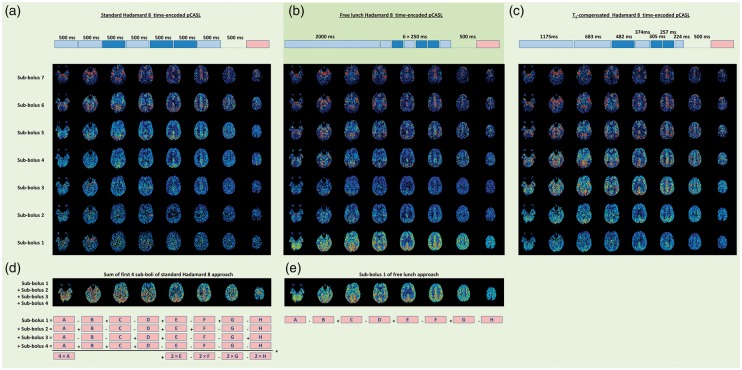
The result of three Hadamard time-encoded, background suppressed pCASL-scans of 5.5 min each (scans acquired on a 3 T MRI scanner (Ingenia, Philips, The Netherlands) on a 43-year-old volunteer who provided informed consent under an approved IRB-protocol; scanner software was locally adapted to allow time-encoded ASL); all sub-boli images have been corrected for T1-decay of label based upon a T1 of 1650 ms. (a) Hadamard-8 matrix with seven blocks of 500 ms each with an additional 500 ms delay before start of the readout module. (b) Hadamard-8 ‘free lunch’ matrix with a perfusion block of 2000 ms and six blocks of 250 ms each with an additional 500 ms delay before start of readout. (c) Hadamard-8 ‘T1-compensated’ matrix with seven blocks of respectively 1175, 683, 482, 374, 305, 257 and 224 ms with an additional 500 ms delay before start of readout. Block duration selected based upon T1-compensation with a T1 of 1650 ms. (d) Result of summing sub-boli 1–4 from the equidistant (traditional) Hadamard-8 matrix (a). (e) The first sub-bolus image of the free lunch approach (b) for comparison purposes.
